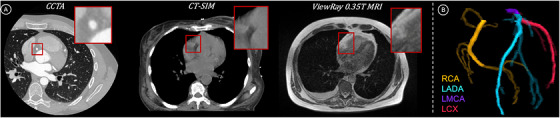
(a) Comparison between contrast‐enhanced CCTA (left), CT‐SIM (middle), and MR‐Linac (right), where the right coronary artery is highlighted with a red box. Coronary arteries are heavily blurred due to cardiac motion in the CT‐SIM/MR‐Linac datasets. (b) Example main‐branch coronary artery delineations based on current RT guidelines (bright) versus the full coronary artery branches delineated on CCTA (dark), where >50% of the right and left anterior descending coronary artery volumes are excluded, highlighting the advantages of an extended model for cardiotoxicity assessment.
